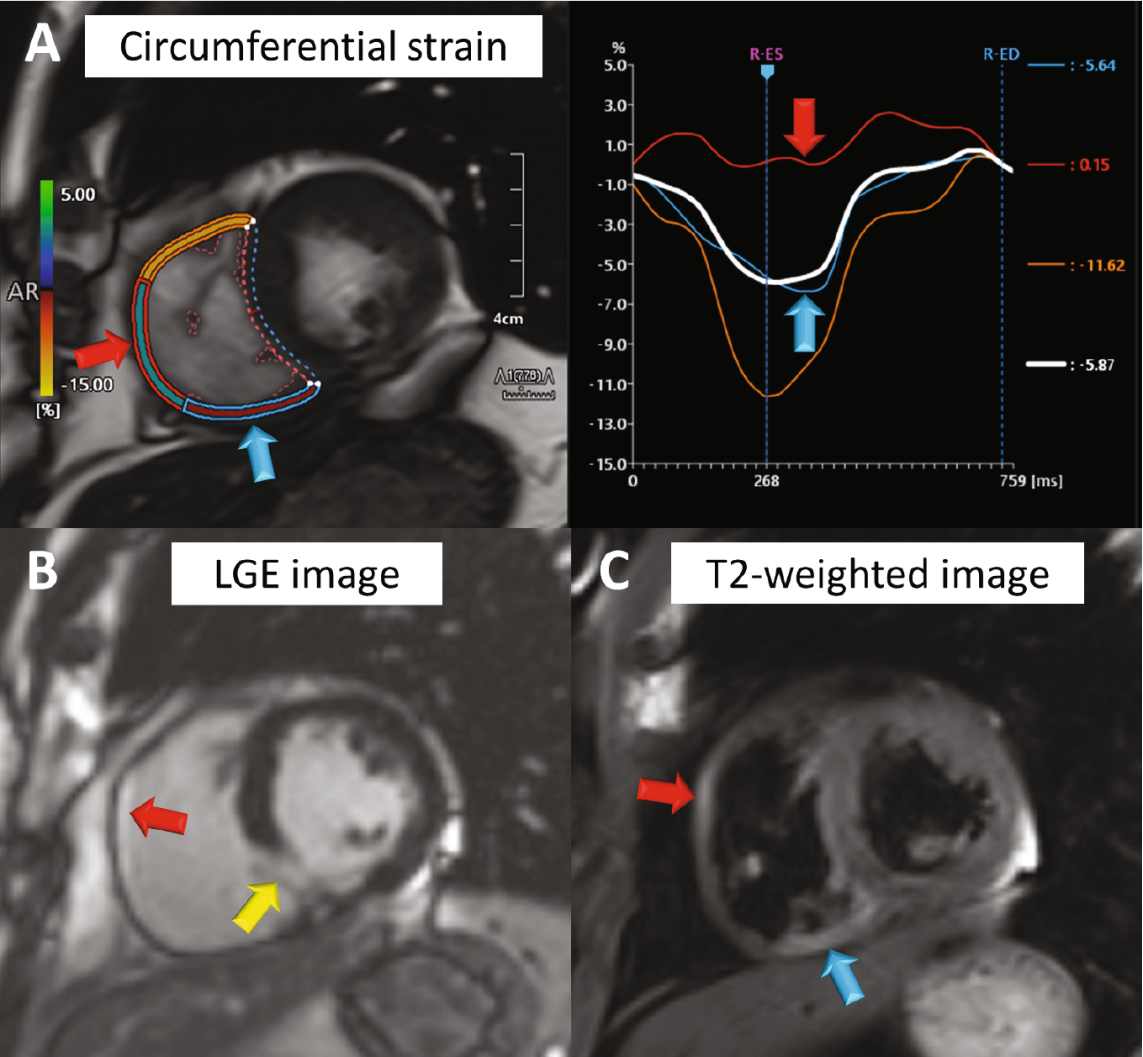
Cardiac magnetic resonance imaging 2 weeks after admission. (A) Reduced circumferential strain is seen in the inferolateral right ventricular free wall (arrows). (B) Late gadolinium enhancement imaging shows the infarcted right ventricular free wall (red arrow) and transmural infarction in the inferoseptal wall (yellow arrow). (C) T2-weighted sequence with fat suppression demonstrates a hyperintense signal of the right ventricular free wall (arrows), suggesting postinfarction inflammation/edema.
